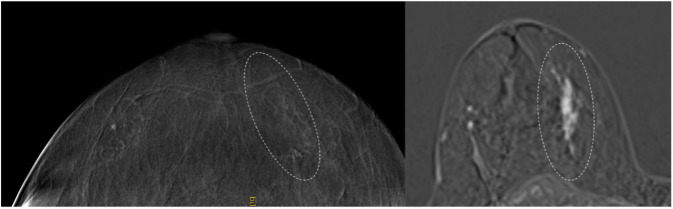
48-year-old woman with segmental pleomorphic calcifications in the medial right breast (DCIS, G3). Segmental heterogeneous non-mass enhancement is clearly visible in CE-MRI (right) with high conspicuity, but conspicuity was low in CEM (left). Note that the recombined CEM image has been mirrored to improve image comparison
